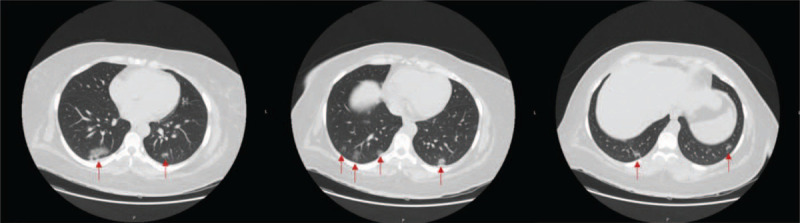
CT Chest shows multifocal lower lung predominant peripheral bronchocentric ground glass opacities (red arrows) compatible with viral/atypical pneumonia.
